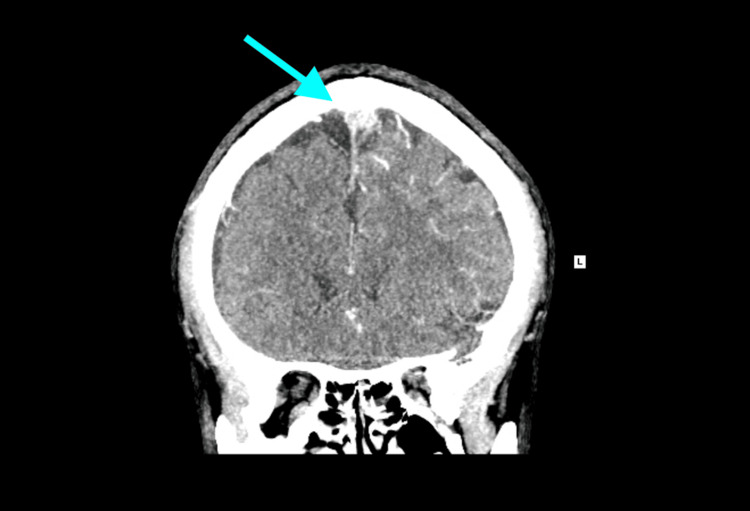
CT venography shows superior sagittal sinus thrombosis (blue arrow)
